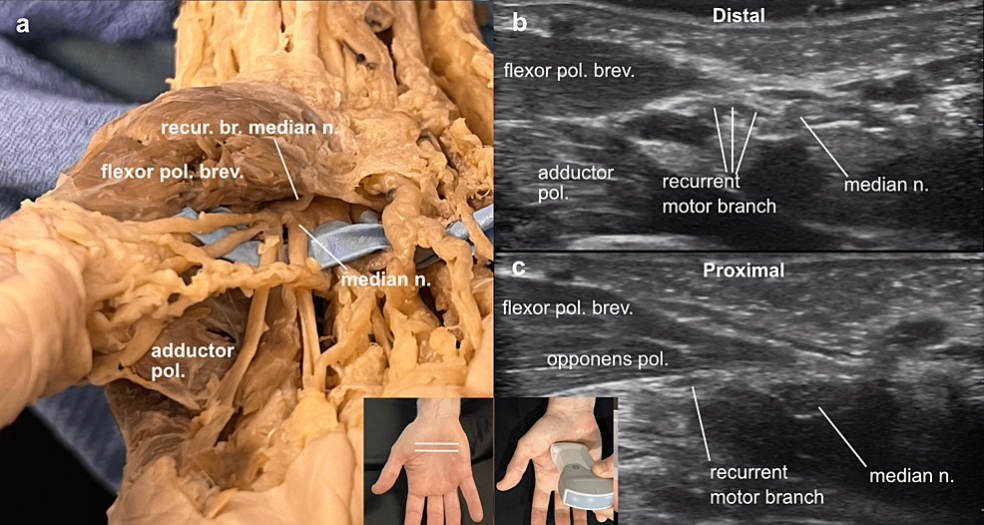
Recurrent motor branch of the median nerve Recurrent motor branch of the median nerve: (a) In the cadaver’s right hand, the recurrent branch was demonstrated looping back from the distal end of the carpal tunnel to innervate the thenar muscles, (b) on ultrasound of a right hand, the probe marker oriented to the right (the thumb), the recurrent branch of the median nerve was followed distal to proximal.
